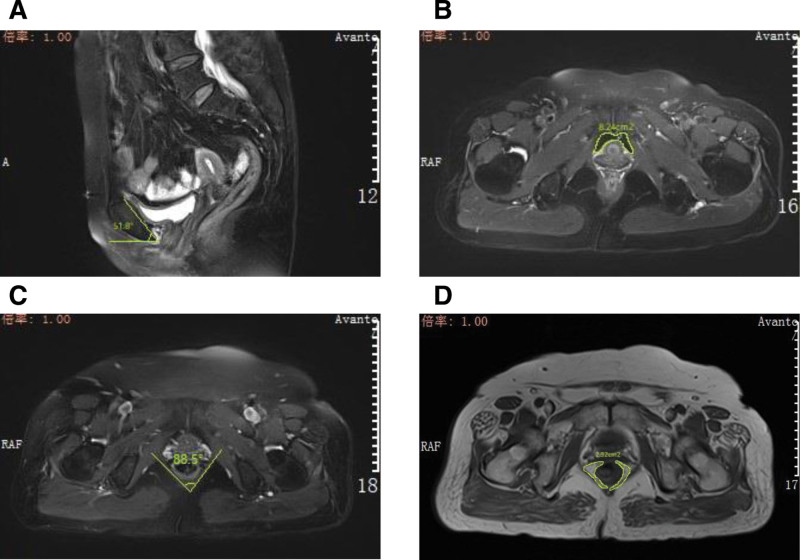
MRI parameters. (A) Pelvic floor MRI, sagittal T1WI sequence image of a 68-year-old woman with a pubic symphysis inclination angle of 51.8°. (B) Pelvic floor MRI with transverse T1WI sequence images of a 66-year-old female with an anterior bladder space area of 8.24 cm^2^. (C) Pelvic floor MRI with a transverse T1WI sequence image of a 67-year-old female with a puborectal muscle pinch angle of 88.5°. (D) Pelvic floor MRI with a transverse compression lipid T2WI sequence image of a 67-year-old female with a rectovaginal-anal levator gap area of 2.32 cm^2^. MRI = magnetic resonance imaging, T1WI = T1-weighted imaging, T2WI = T2-weighted imaging.
